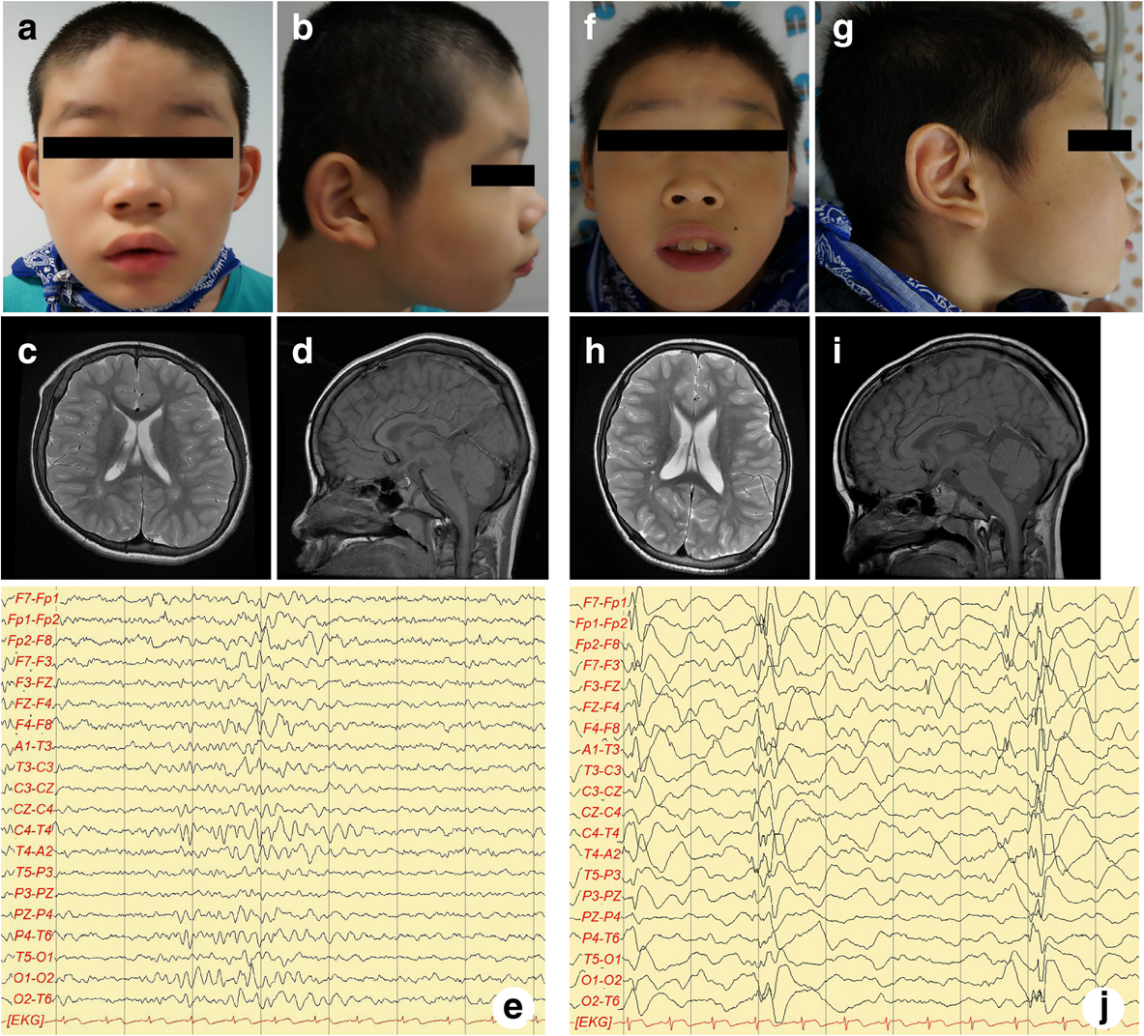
Clinical features of patient 1 and 2. Clinical features of patient 1 (a-e). Facial dysmorphisms with brachycephaly, slightly upturned nares, large and low set ears were observed (a, b). Axial T2 (c) and sagital T1 (d)-weighted brain MRI images were in normal limits. interictal EEG shows epileptic sharp wave discharges from the right temporal cerebral area with poorly regulated posterior rhythm and slow background activity (e). Clinical features of patient 2 (f-j). Facial dysmorphisms with large ears, slightly upturned nares, and midface hypoplasia followed by depressed nasal bridge were observed (f, g). Axial T2 (h) and sagital T1 (i)-weighted brain MRI images showed no abnormalities. interictal-EEG reveals frequent generalized burst of epileptic sharp wave discharges from the both frontal cerebral area followed by attenuation of background activity (j)
